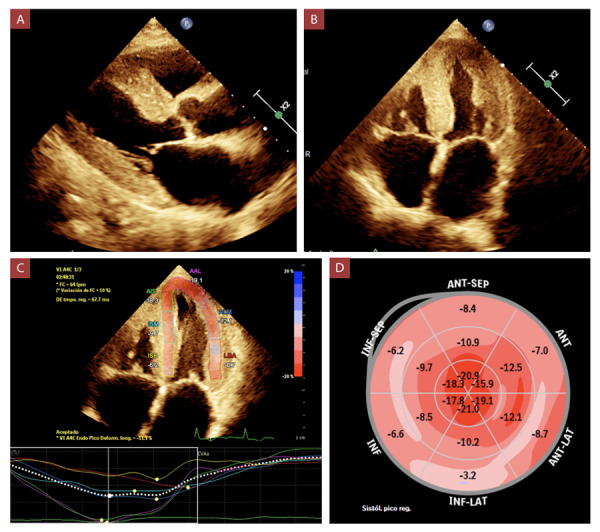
Ecocardiograma transtorácico correspondiente al caso 6. A) Vista paraesternal eje largo que muestra engrosamiento del septum interventricular y pared posterior del ventrículo izquierdo. B) Vista apical 4-cámaras que muestra engrosamiento de las paredes de ambos ventrículos, del septum interventricular e interatrial, dilatación biatrial, y engrosamiento de los velos de las válvulas mitral y tricúspide. C) Strain longitudinal global reducido (-11), con valores conservados en ápex y reducción a nivel basal y medio. D) Mapa polar que muestra todos los segmentos del ventrículo izquierdo con patrón característico en “ojo de buey”.
